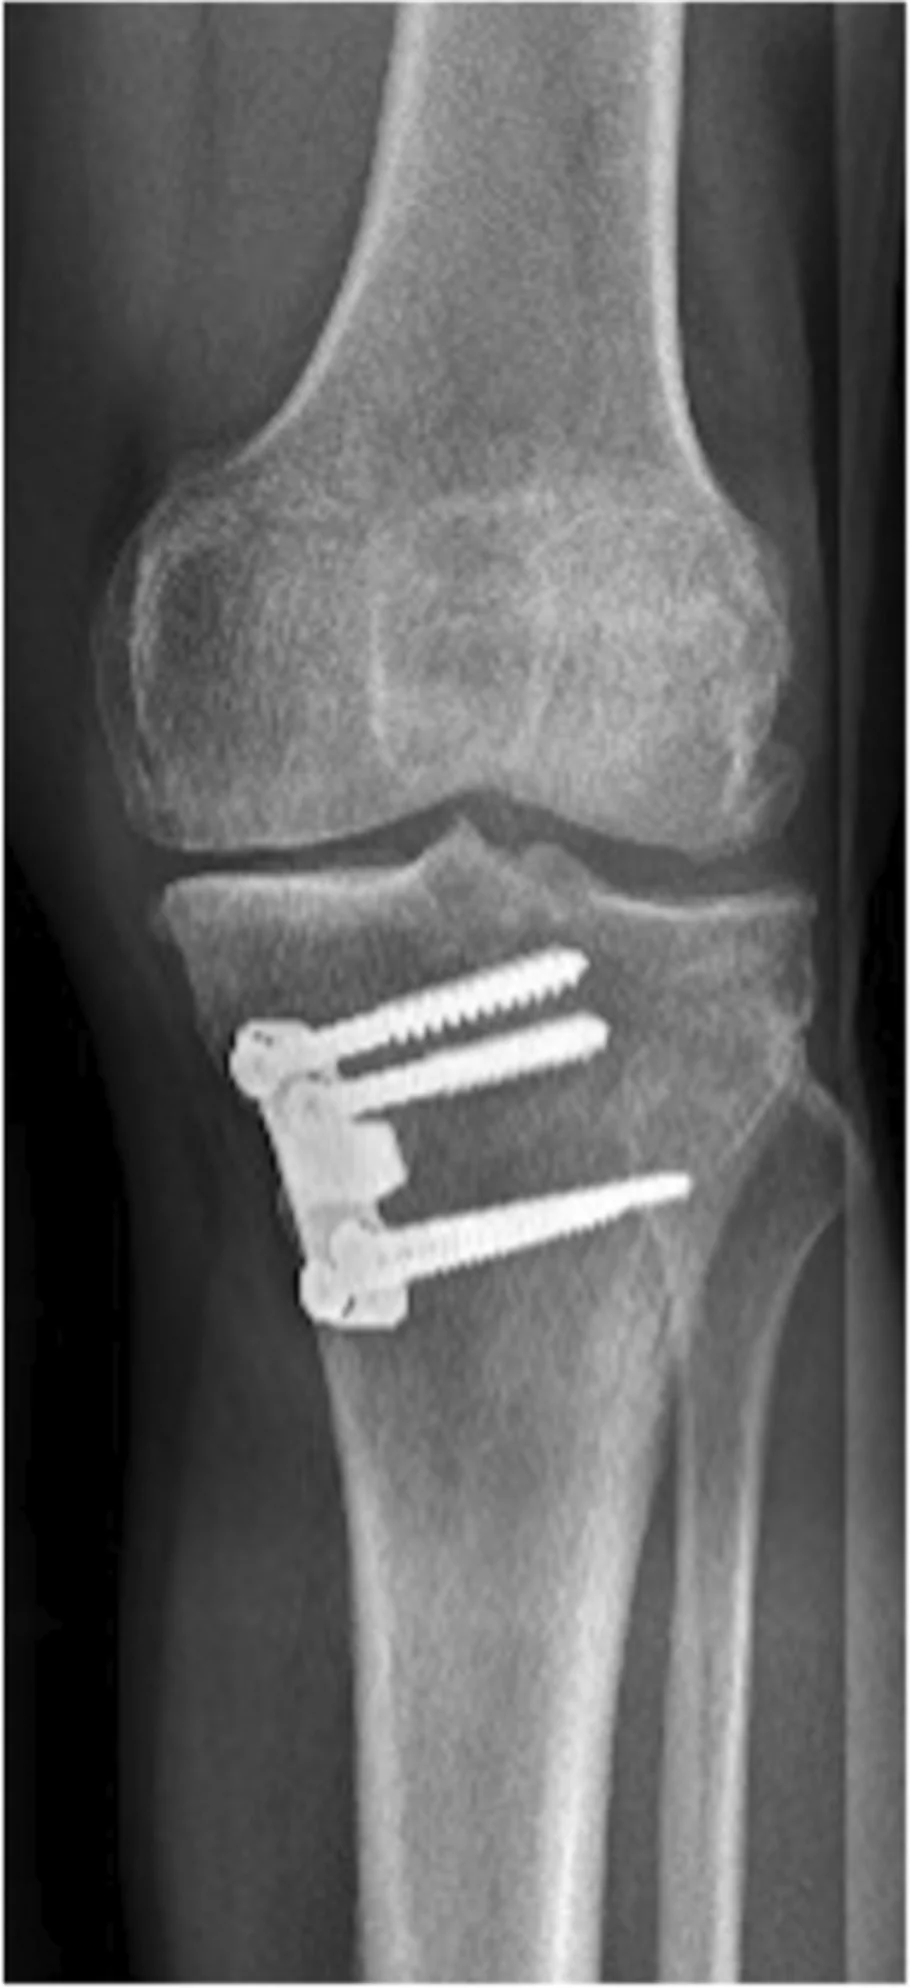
Postoperative radiograph of a high tibial osteotomy fixed with a Puddu plate at 8‐year follow‐up.
